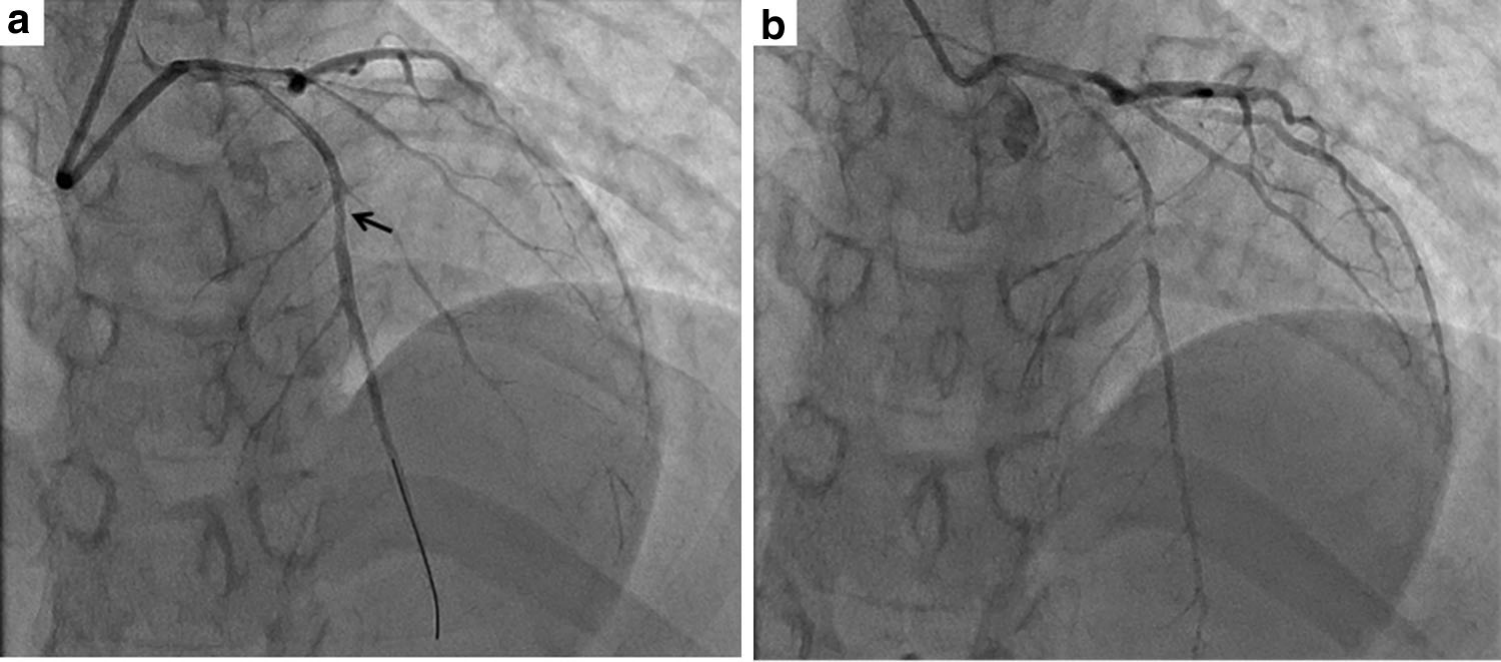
Angiogram of the left coronary artery before and after the intravascular ultrasound (IVUS) examination. a After IVUS, the stenosis in the left anterior descending artery improved slightly (*arrow*), despite the insertion of a guide-wire. b Before the IVUS, see legend of Fig. 2a
